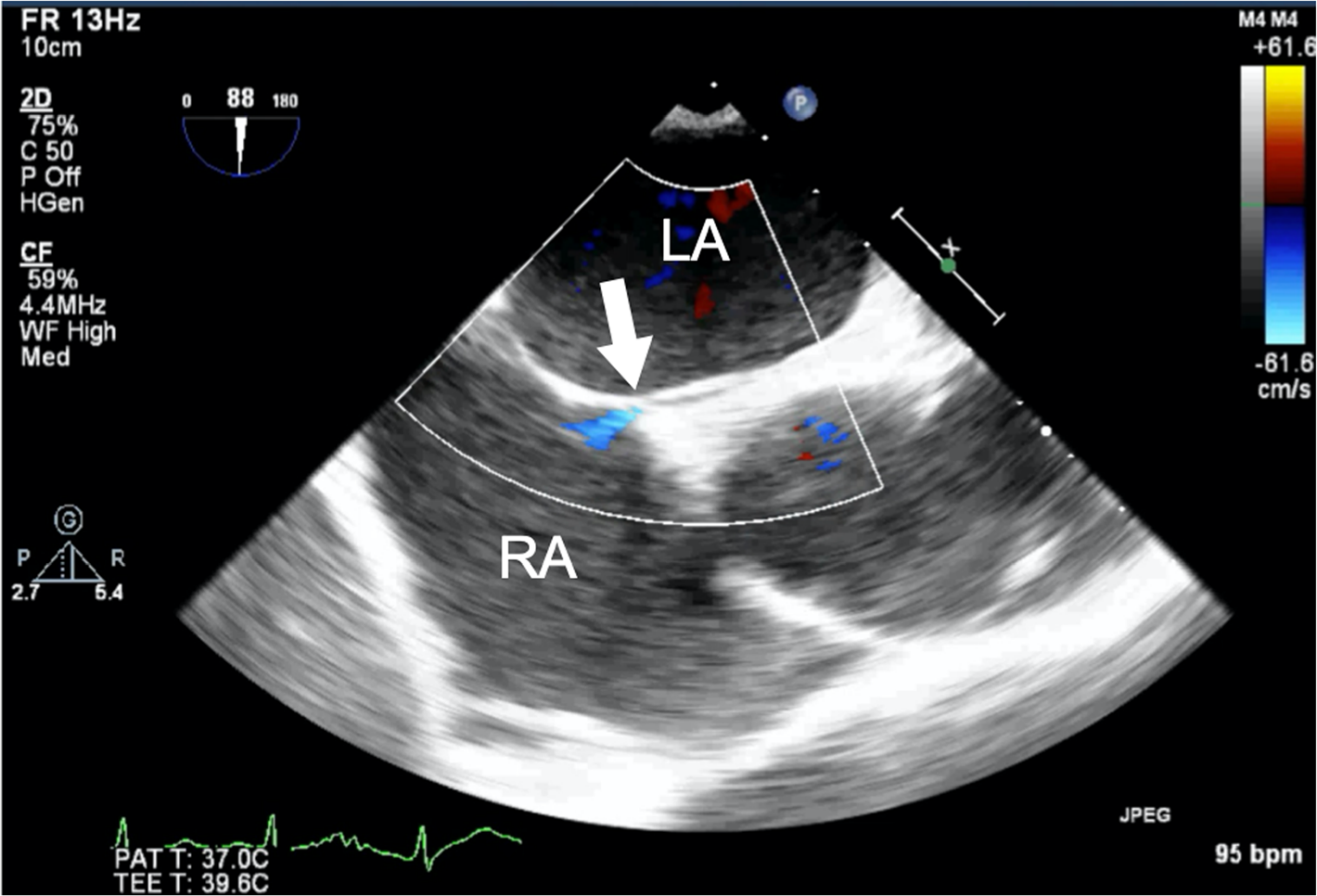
Transesophageal echocardiography showing a patent foramen ovale and shunt flow (arrow). LA: left atrium, RA: right atrium
